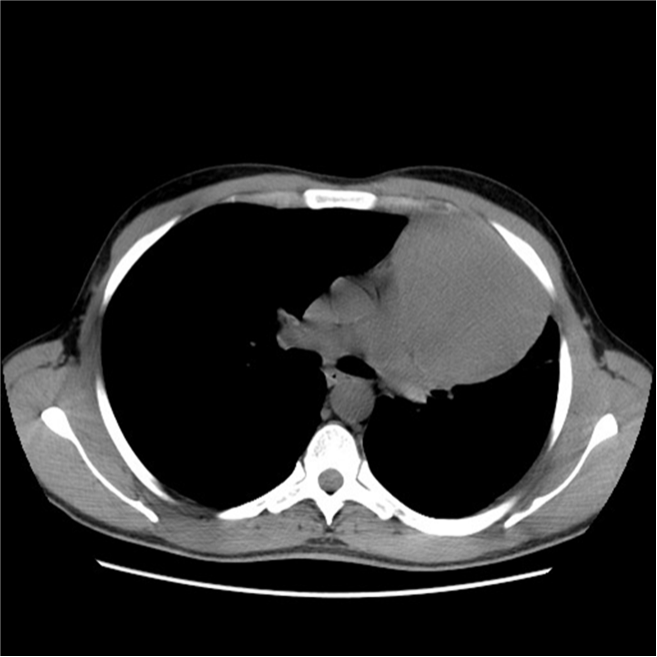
Computed tomography scan of the chest: partial response after 4 cycles of chemotherapy.
